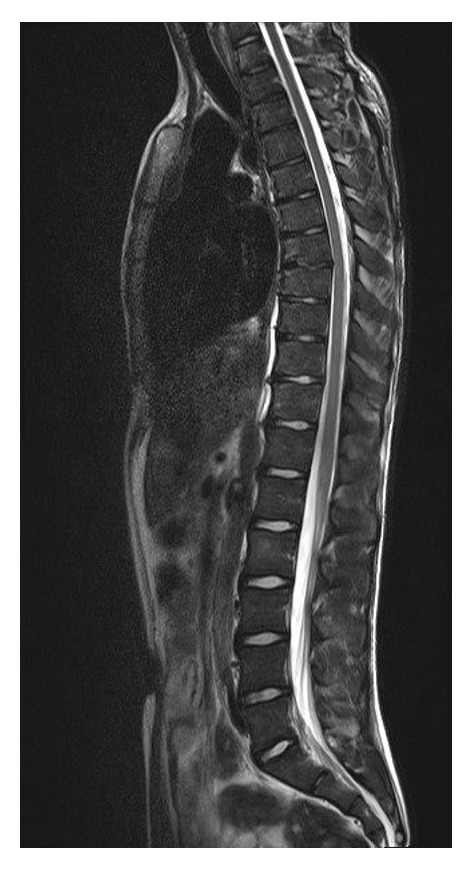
MRI of the spine, showing a fracture of the base plate in thoracic spine body 7, such as lesions in lumbar spine body 2.
